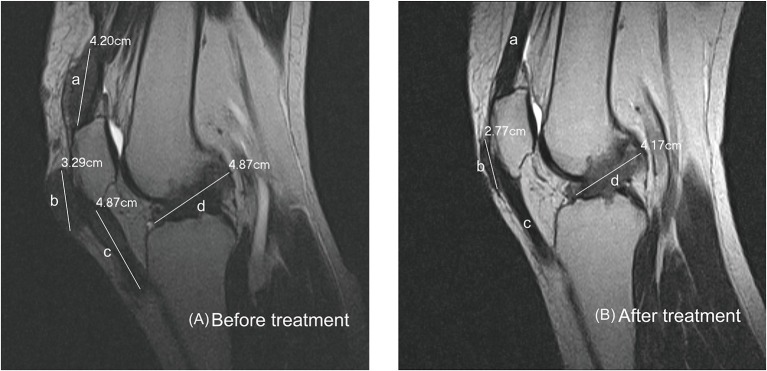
Intra-articular tophi evaluation and response assessment. (A) Baseline MRI of the knee joint of a 31-year-old man with subcutaneous tophaceous gout revealed multiple intra-articular tophi. A tophus within the knee joint was measured according to response evaluation criteria in solid tumors criteria. The total length in this plane is the sum of *a* + *b* + *c* + *d*, 17.23 cm. (B) Follow-up MRI revealed decreased intra-articular tophi size. The tophi at *a* and *c* dissolved, and those at *b* and *d* decreased.
